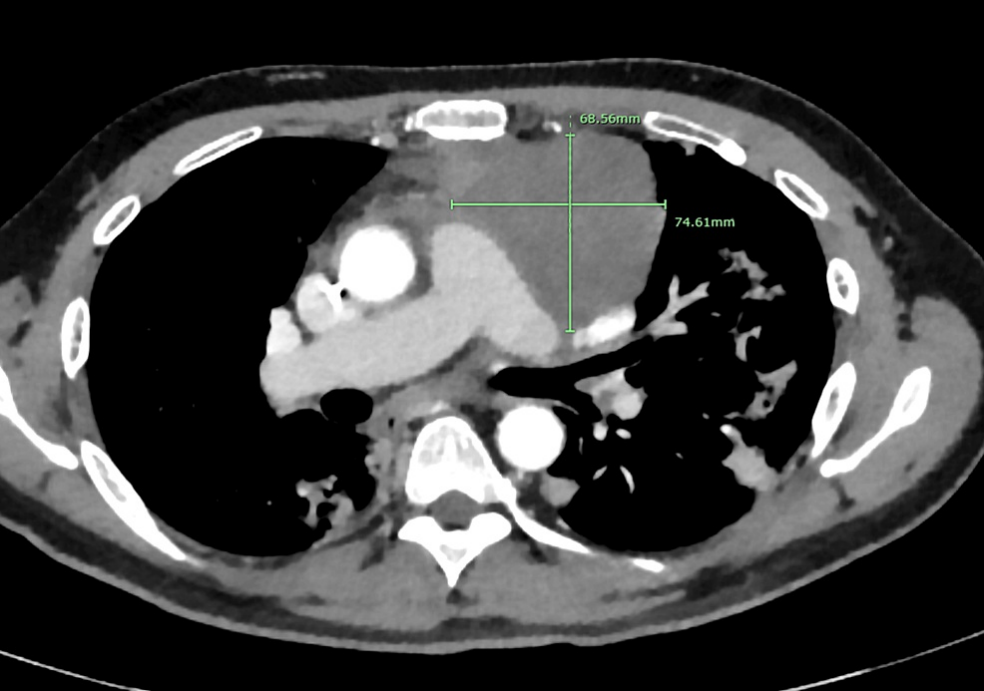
Post-treatment CT scan of the chest with IV contrast showing reduced size of the mass.
